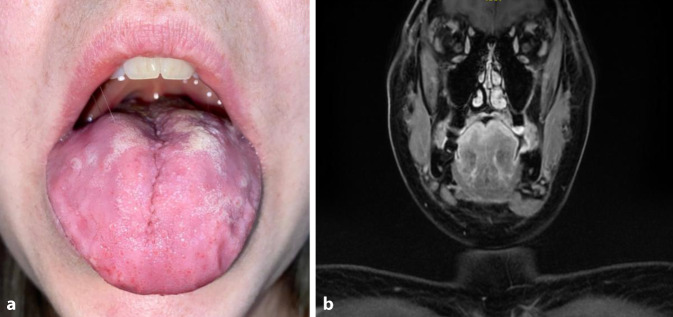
a Physical examination with pronounced swelling of the tongue at the initial presentation. b T1-weighted magnetic resonance imaging (MRI) with gadolinium: Coronal section showing strong contrast enhancement of the upper third of the tongue including the tongue surface
